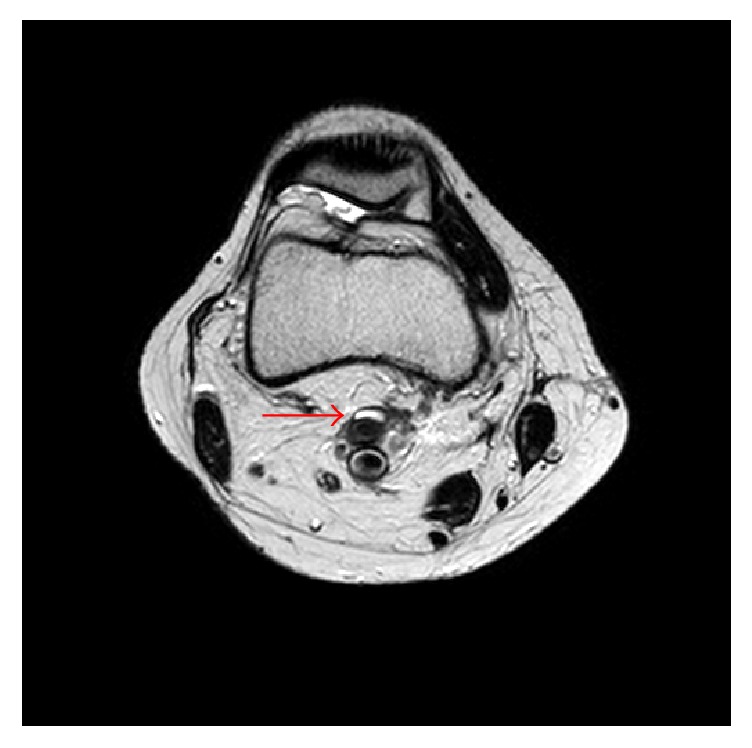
Postoperative MRI revealed 5 square mm of high intensity adventitial area (arrow) that was suspected as remaining cystic lesion.
